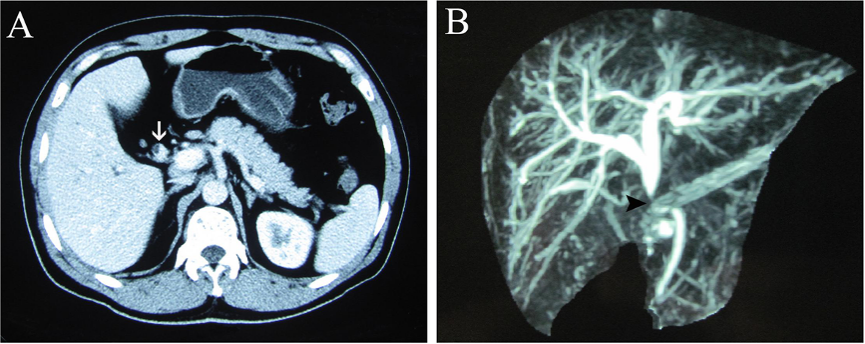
Preoperative imaging examination. (A) Computed tomography showed biliary stricture (white arrow). (B) Magnetic resonance cholangiopancreatography revealed biliary stricture (black arrow) and dilatation at the top of the stricture.
